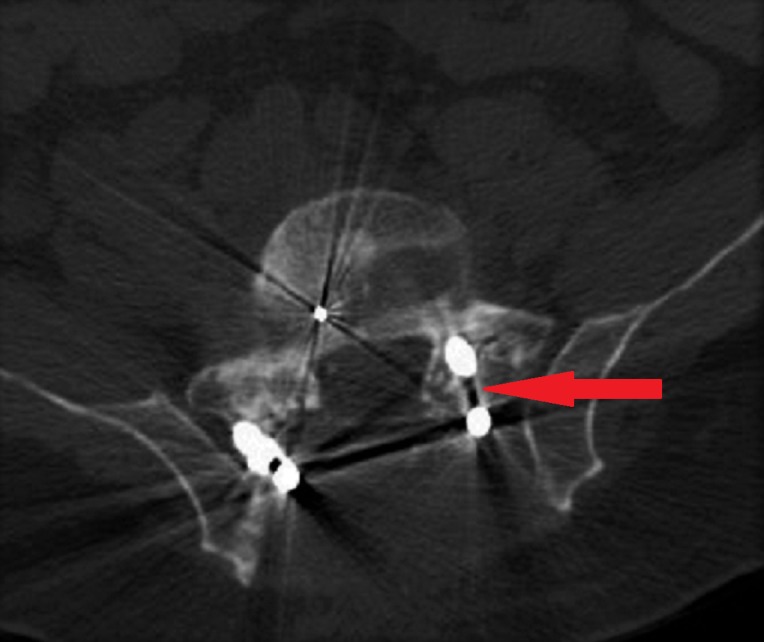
Computed tomography (CT) scan of fluoroscopy-guided hand-placed screws demonstrates the only Grade-E accuracy for screw placement (Right screw) as denoted by arrow at S1 level.
